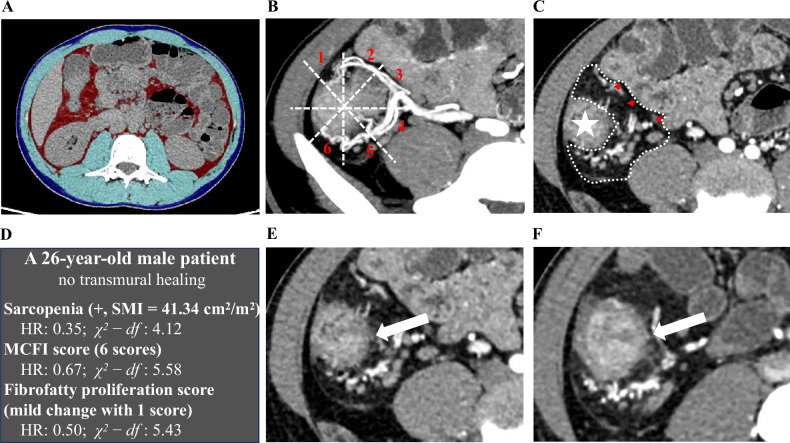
Another patient who experienced a failure to achieve TH (A–F). A Axial non-contrast CT image at the L3 level shows the segmentation of skeletal muscle (light blue), visceral adipose tissue (red), and subcutaneous adipose tissue (dark blue). The patient was diagnosed with sarcopenia, with an SMI of 41.34 cm^2^/m^2^. B, C The MCFI and fibrofatty proliferation score of the ascending colon (indicated by the star) are scored as 6 and 1, respectively. E Axial post-contrast CT image shows the pretreatment inflammation of the ascending colon (arrow). F After 62 weeks of UST treatment, this patient experienced a failure to achieve TH, with BWT > 3 mm, mural hyperenhancement, and mesenteric edema. TH, transmural healing; L3, third lumbar vertebra; MCFI, mesenteric creeping fat index; SMI, skeletal muscle index; UST, ustekinumab; BWT, bowel wall thickness
